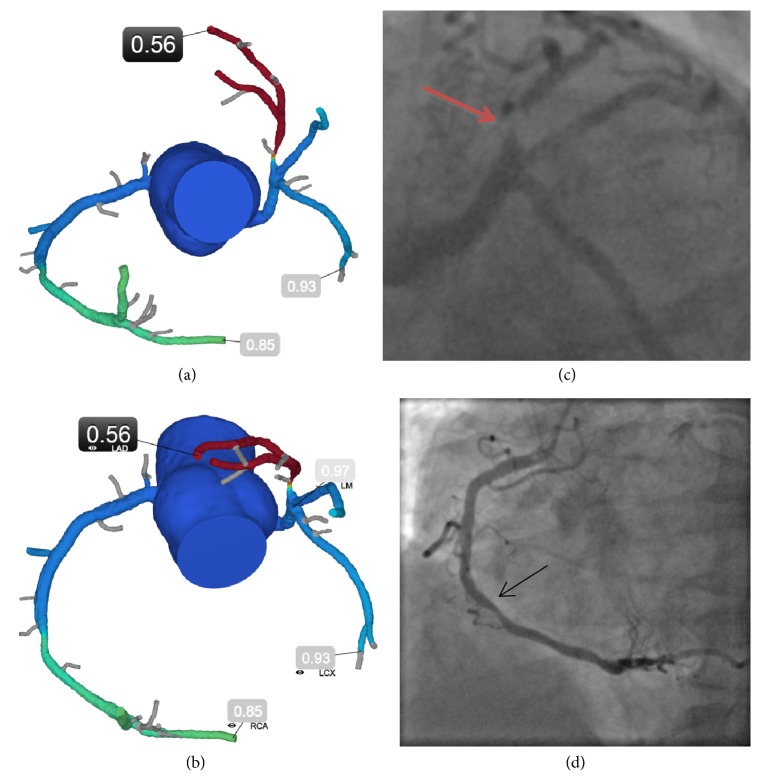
FFR_CT_ redefining revascularization strategy. A 68-year-old male with tobacco abuse, hypertension, hyperlipidemia, diabetes, and shortness of breath underwent coronary CTA demonstrating a 70%-90% stenosis of the proximal LAD and a 50%-70% stenosis of the mid-RCA. The initial decision based on the coronary anatomy alone was to refer the patient for coronary artery bypass graft surgery. However, FFR_CT_ was performed to help inform the invasive procedure. FFR_CT_ distal to the proximal LAD and mid-RCA stenoses were 0.56 and 0.85, respectively. The patient was rescheduled for PCI, received one stent in the proximal LAD, and is asymptomatic at three-year follow-up. Teaching points: with the functionally significant stenosis in the proximal LAD supplying a large territory of myocardium and his continued symptoms on optimal medical therapy, the patient was taken to the catheterization laboratory where a drug-eluting stent was placed. In addition, the cardiologist performed invasive FFR for the moderate stenosis in the RCA which was 0.86, corroborating the nonfunctionally significant lesion and no intervention was performed. This case highlights the unique opportunity to noninvasively provide physiological information on a per-lesion level. This enables a more informed decision around recommendations for ICA, specifically about which vessels to further interrogate and may redefine revascularization strategy. Even when the decision on referral to ICA is already taken because of symptoms and high-risk anatomy as determined by coronary CTA, FFR_CT_ may be of relevance by guiding decisions about other intermediate range lesions. FFR_CT_ (a,b) and ICA (c,d). LAD demonstrates a focal proximal severe stenosis (red arrow) that is hemodynamically significant. RCA demonstrates a focal mid moderate stenosis (black arrow) that is not hemodynamically significant. FFR_CT_ indicates fractional flow reserve derived from coronary computed tomography angiography (CTA) datasets; ICA, invasive coronary angiography; LAD, left anterior descending artery; and RCA, right coronary artery.
